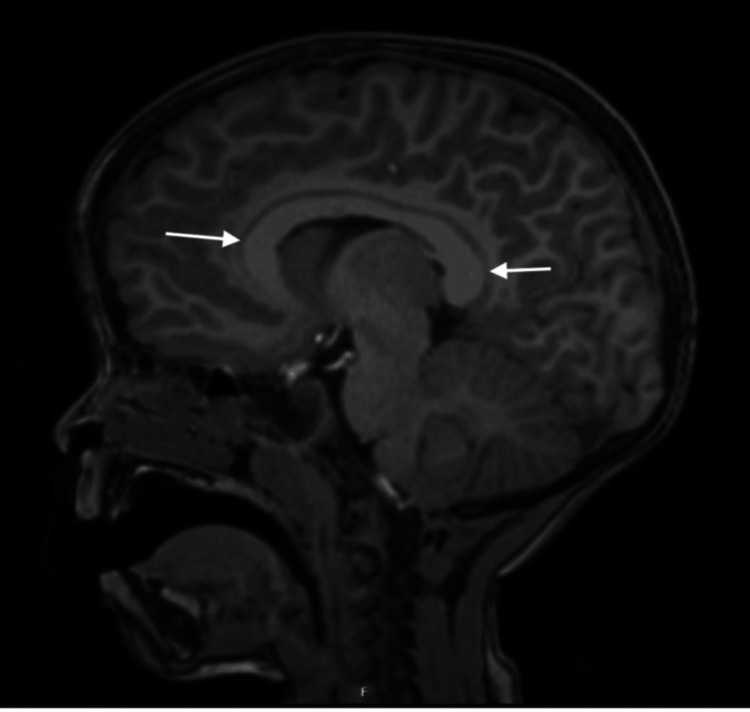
Sagittal T1-weighted brain MRI showing a diffusely thickened corpus callosum (arrows). The corpus callosum appears globally enlarged, a feature described in *PTEN*-related megalencephaly.
